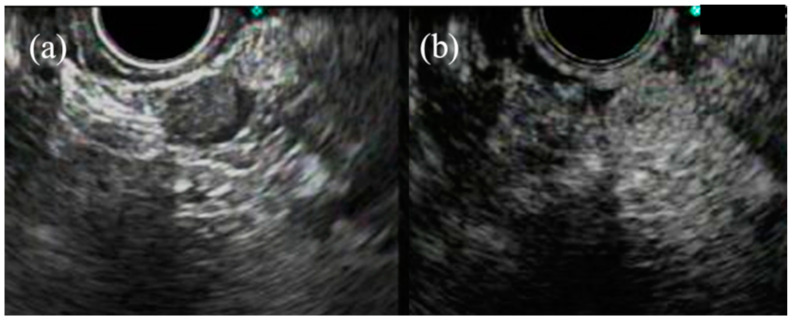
A typical example of a pancreatic neuroendocrine tumor with hyperenhancement. Fundamental B-mode endoscopic ultrasound (EUS) (a) showing a hypo-isoechoic tumor at the pancreatic head, and contrast-harmonic EUS (b) showing a tumor with hyperenhancement.
